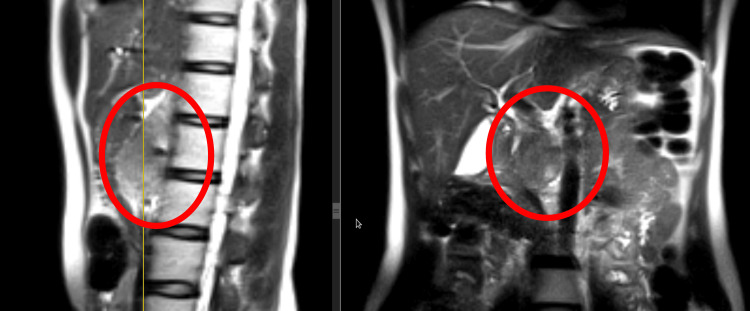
MRI of the chest, sagittal and coronal STIR sequences, demonstrating a heterogeneous posterior mediastinal tumor. STIR: short tau inversion recovery
